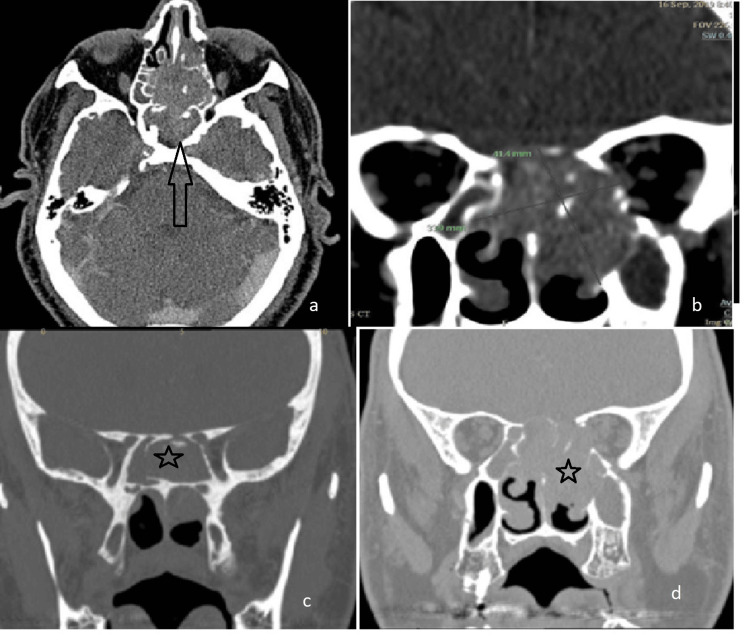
CT scan of sphenoid IP with SCC transformation (a) axial view, (b,c,d) coronal view. IP: inverted papilloma, SCC: squamous cell carcinoma.
